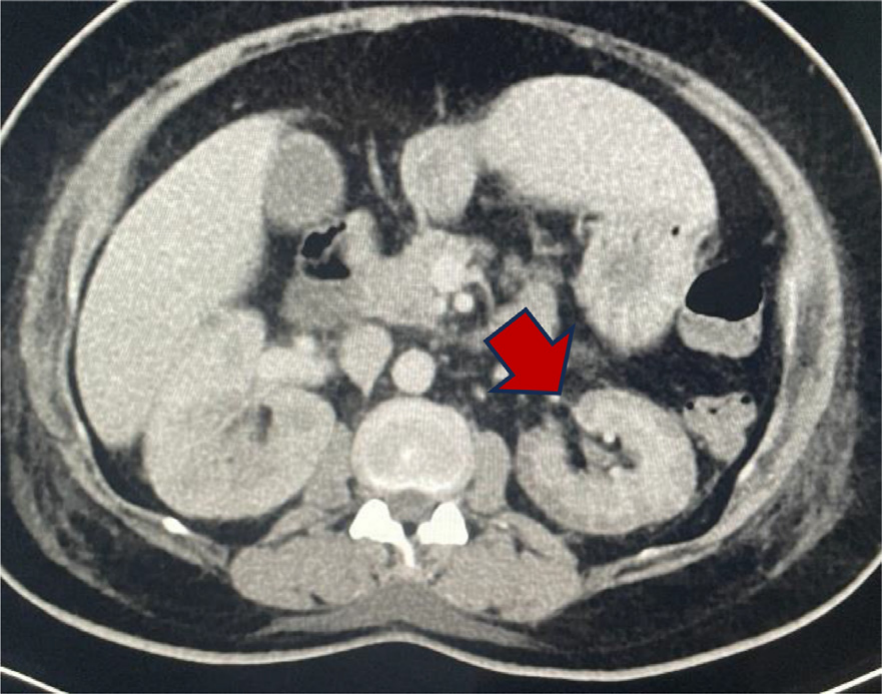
Imaging modality: contrast enhanced computed tomography of the abdomen. Findings: There is diffuse cortical hypoenhancement of the left renal cortex with normal enhancement of the medulla. This is due to renal cortical necrosis.
